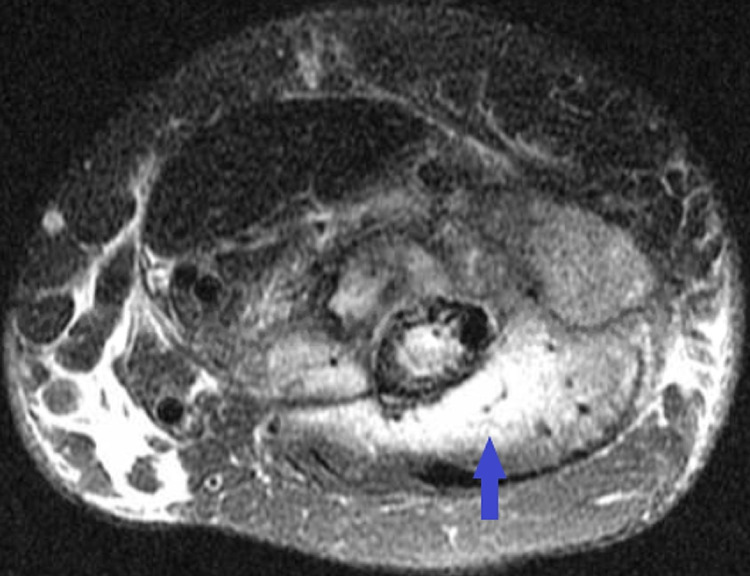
MRI of the left upper extremity showing showed left humerus and proximal radius osteomyelitis, myositis of brachialis, brachioradialis, and triceps muscles (blue arrow), complex glenohumeral joint effusion, and biceps tenosynovitis MRI: Magnetic Resonance Imaging
